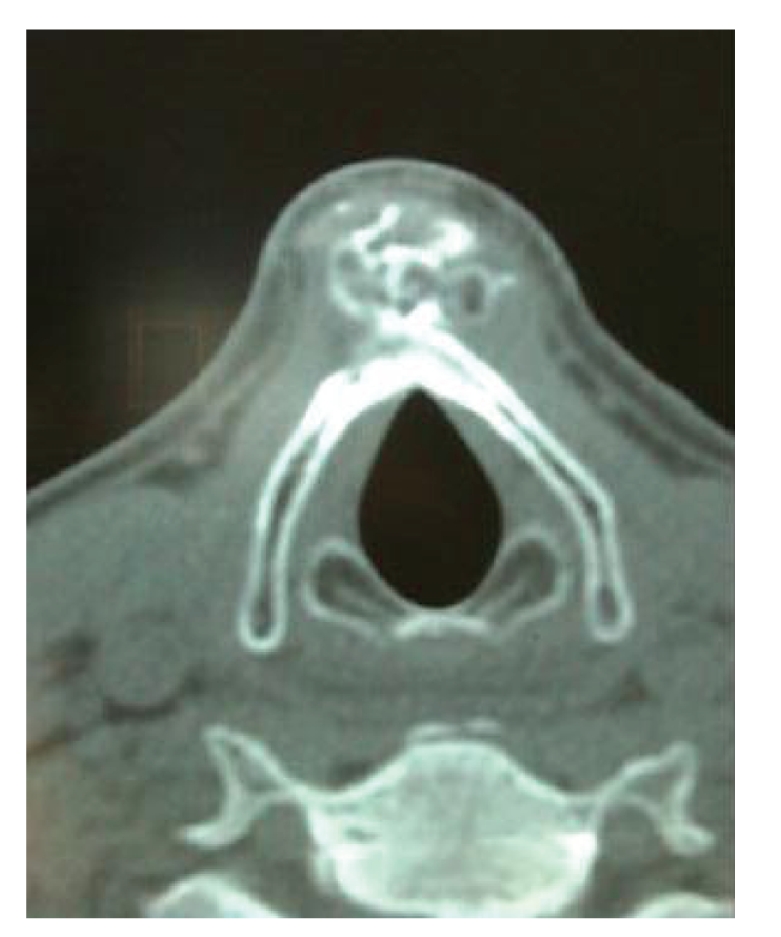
CT image of the laryngeal chondrosarcoma. This shows the lesion originating from the outer cortex with calcifications and ossifications.
